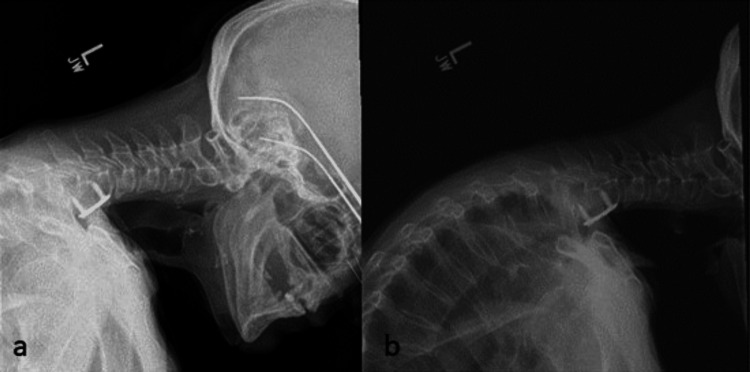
(a) X-ray of patient in relaxed lateral position for initial clinic X-rays six weeks post DHS onset; (b) X-ray of patient in extension for initial clinic visit six weeks post DHS onset.
